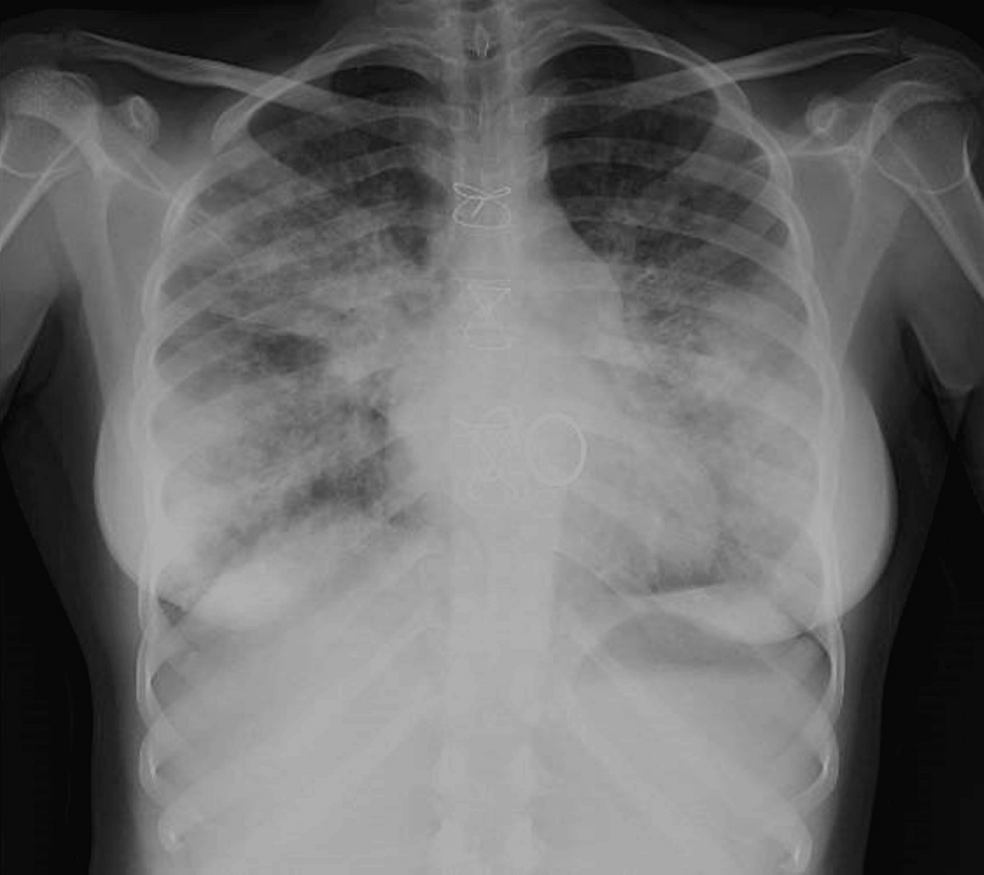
Thoracic X-ray on admission Thoracic X-ray with bilateral alveolar infiltrates.
